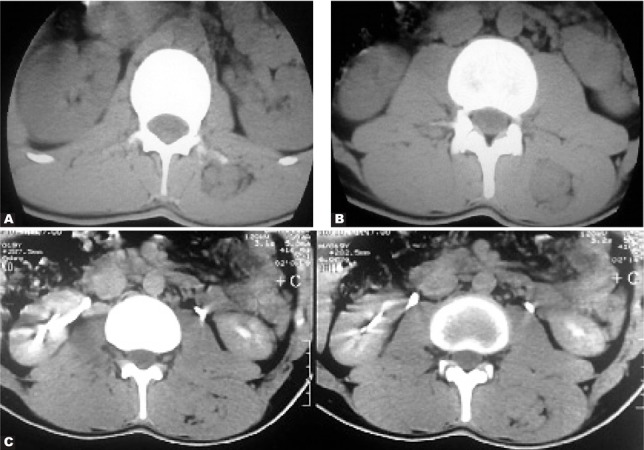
A 19-year-old man with 6-month pain on the left side of the thoraco-lumbar area. A and B.Axial CTscan without IV contrast C.Axial CT scan after contrast injection
